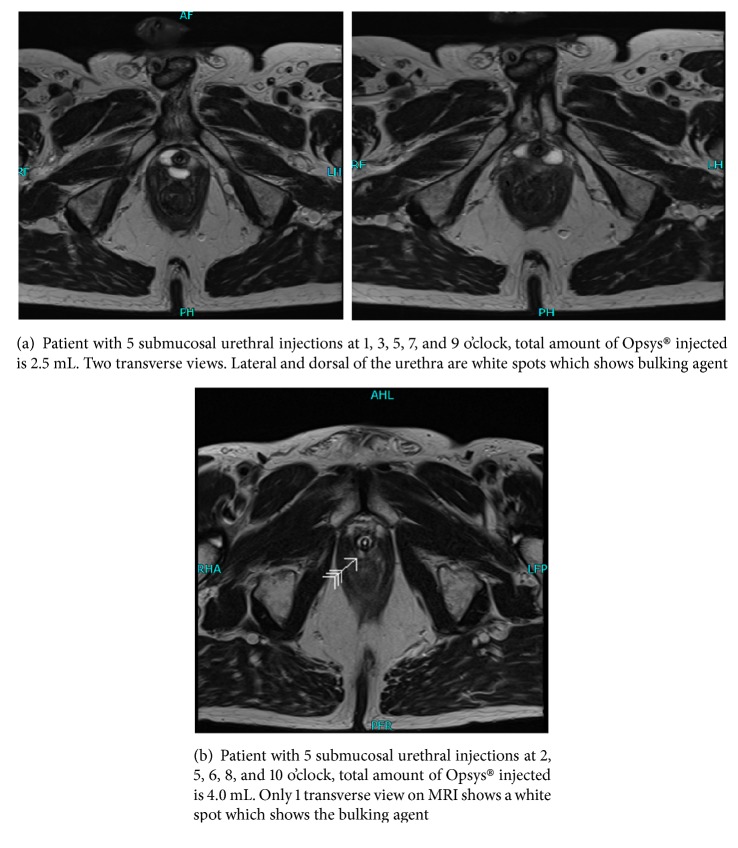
Magnetic resonance imaging (MRI
